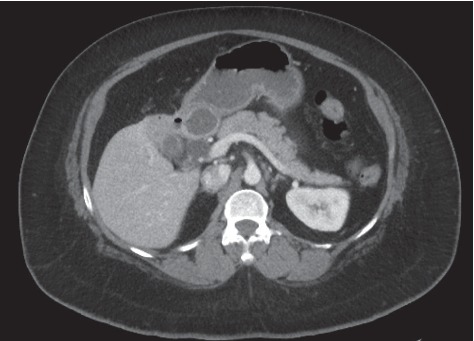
CT scan showing a large stone in the duodenum and a cholecystoduodenal fistula.
